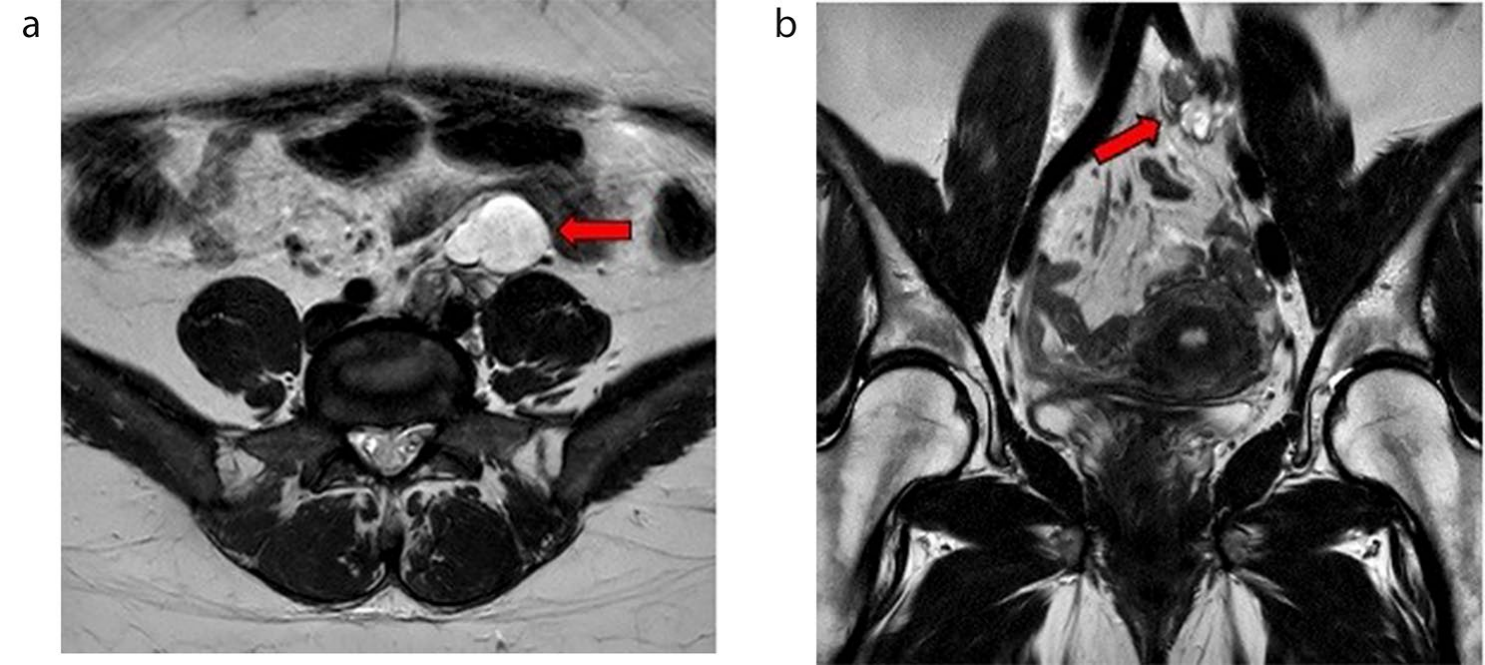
Abdominal MRI images in T2W/TSE-tra (a) and cor (b) showing a multicystic solid retroperitoneal mass ventral to the psoas muscle and the left iliac artery (red arrow) b lobulated, partly cystic and partly solid portion with vascular contact (red arrow)
